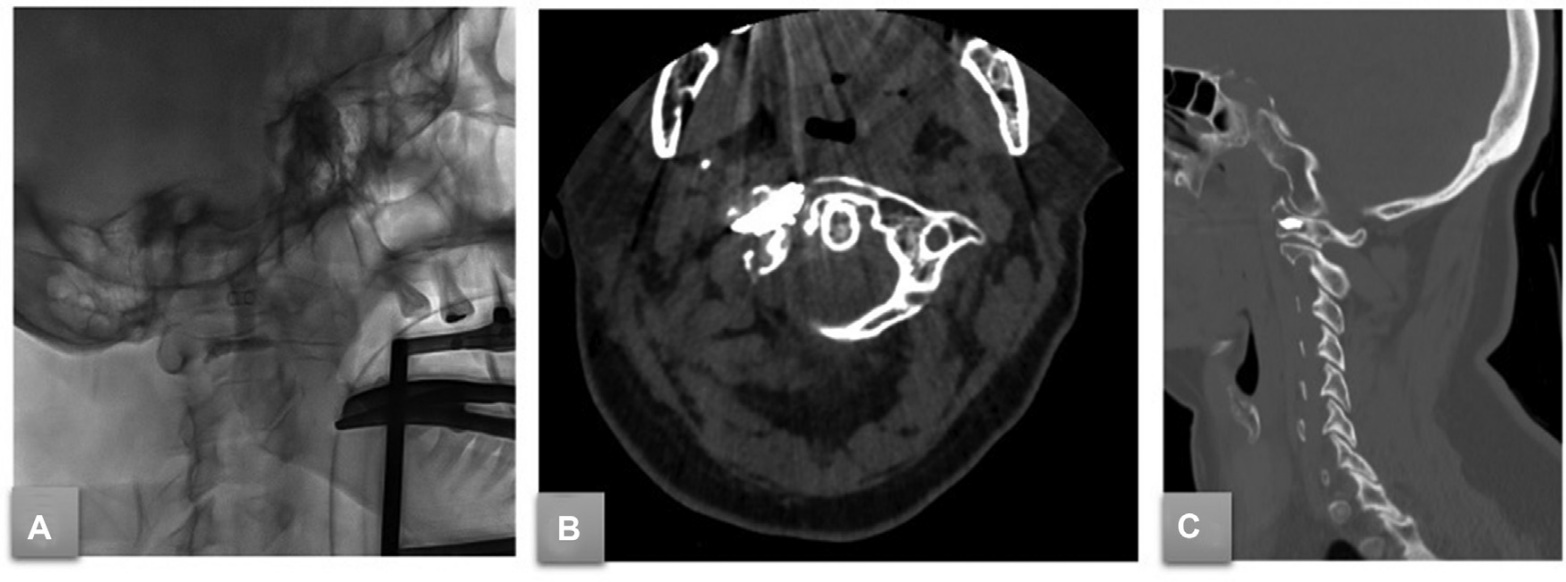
(A) Sagittal fluoroscopic post-procedural imaging after cementoplasty procedure. A 6-month postprocedural axial (B) and sagittal (C) CT scan after high-viscosity bone cement (StabiliT) injection.
